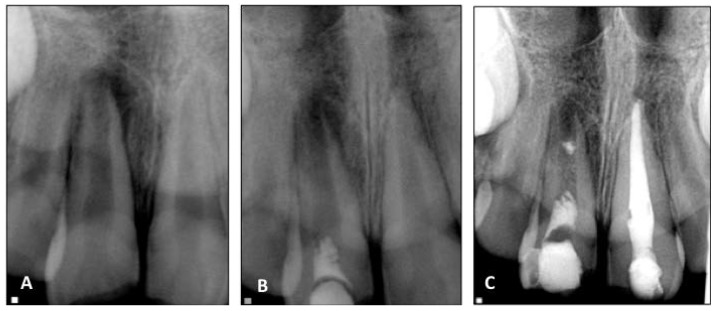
Radiographs of revascularized human immature permanent tooth #8. (A) Preoperative radiograph to show inflammatory periapical lesion; (B) Postoperative radiograph after regenerative endodontic procedures; (C) At 12-month follow-up, no thickening of the canal walls and no continued root maturation are seen.
